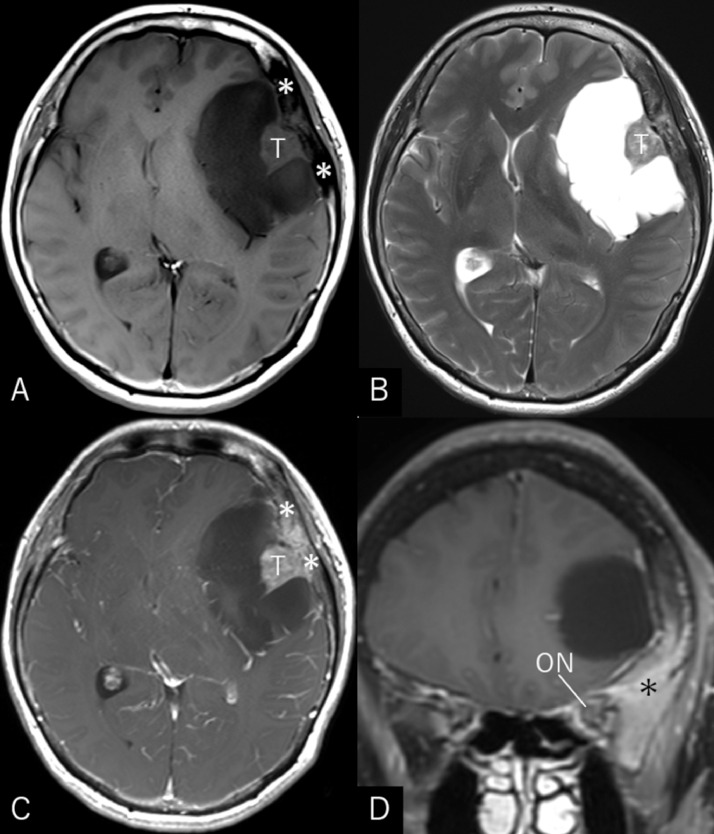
Axial T1- (A), T2-weighted (B) magnetic resonance (MR) images showing a well-demarcated, apparently extra-axial cyst 68 × 46 × 48 mm in maximal dimensions and involving a tumor (*T*) 16 × 20 × 24 mm in maximal dimensions. The tumor shows accompanying cyst components and is attached to the dura mater of the pterional region. Post-contrast axial (C) and coronal (D) MR images showing inhomogeneous enhancement of the tumor and adjacent diploe with extensive involvement of the frontal and sphenoid bones (*asterisk*), while the wall of the cyst is not enhanced. *ON:* optic nerve.
